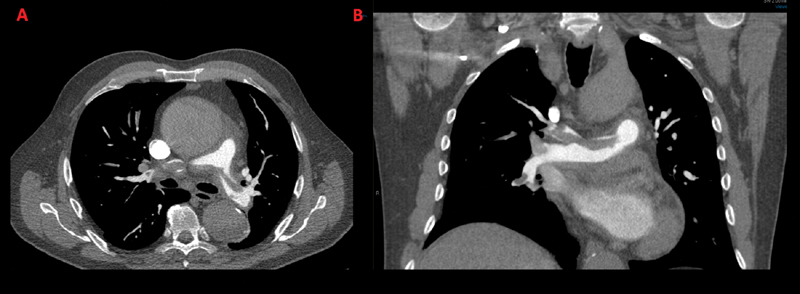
Computed tomography pulmonary angiogram showing large saddle pulmonary embolism in axial (
